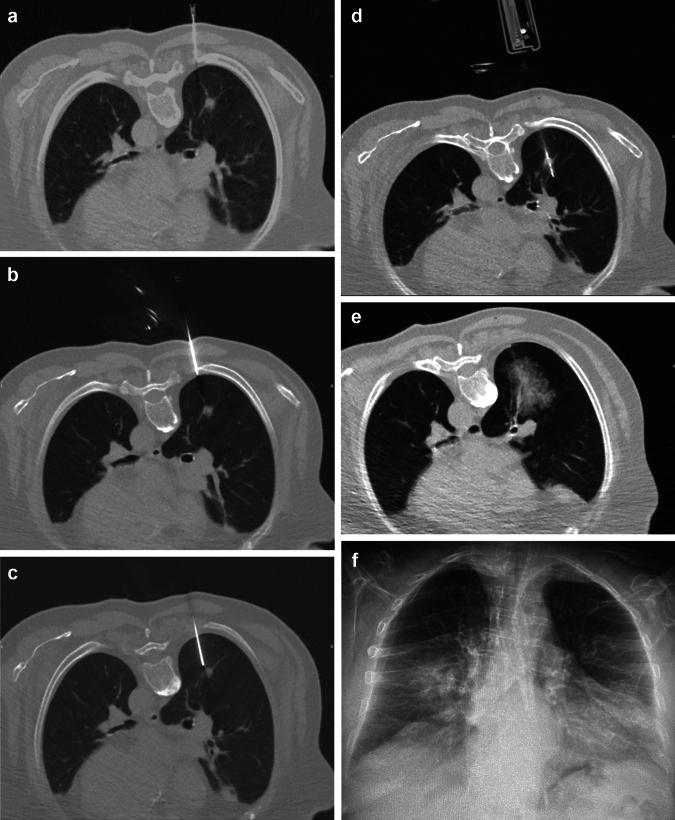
a Local anesthesia performed from the subcutaneous layers to the extra-pleural space. b Coaxial needle is positioned outside the pleura prior to advancing it as close to the nodule as possible. c Coaxial needle pierces the lung in one step and is positioned in the vicinity of the nodule. d The automatic ACECUT biopsy gun is inserted through the coaxial needle and is fired for material retrieval. e CT scan after the biopsy documents hematic alveolar hemorrhage due to the central position of the nodule in communication with larger pulmonary vessels. No pneumothorax is documented. Patient spontaneously resolved hemoptysis 1 h after the procedure. f X-ray at 3 h post the procedure confirms the absence of pneumothorax with medio-basal opacity consistent with alveolar hemorrhage. No pleural effusion is seen.
